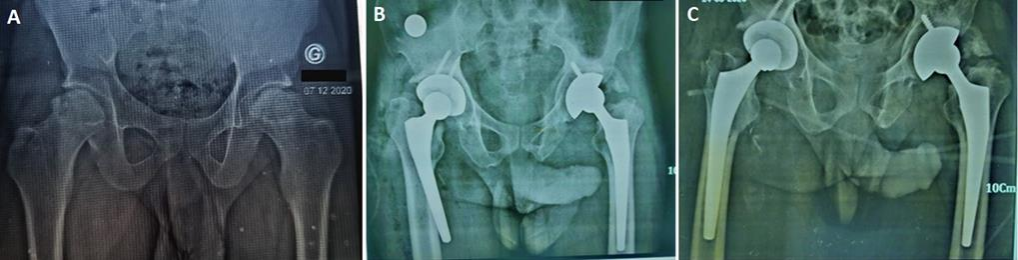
intraoperative complications: A) preoperative X-ray; B) femoral stem malpositioning on the right; C) surgical revision to reposition the femoral stem, Maroua Military Hospital, Cameroon, 2024
